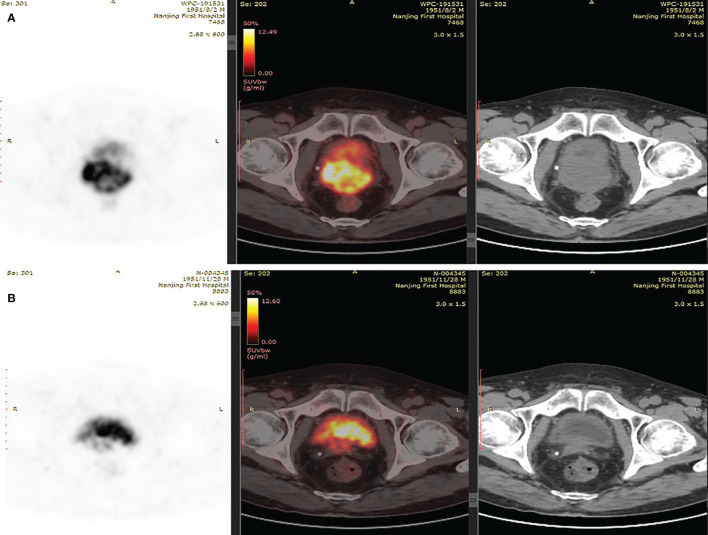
(A) PSMA-PET/CT scans suggested of uneven increase in PSMA expression of prostate. (B) PSMA-PET/CT scans showed shrunken lesions after platinum-based neoadjuvant chemotherapy.
